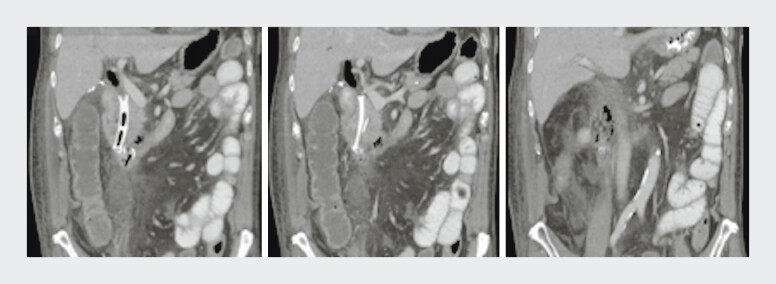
Computed tomography demonstrating small locules of retroperitoneal gas, fast stranding, and a small volume of extraluminal fluid.
